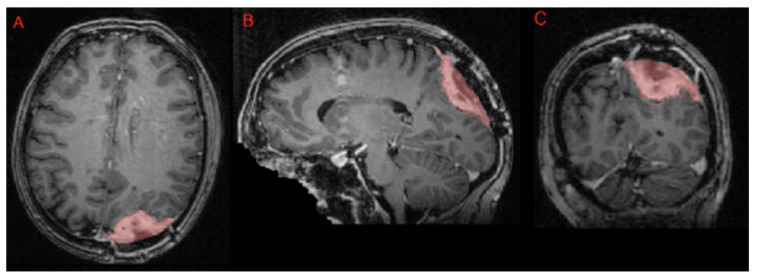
Brain MRI of a patient showing a postoperative left parietal meningioma target volume (red), as delineated by the treating institution, presented in different planes: Axial view in panel (A), sagittal view in panel (B), and coronal view in panel (C). Figure adapted and modified from: [33], licensed under the CC BY 4.0: https://creativecommons.org/licenses/by/4.0/ (accessed 12 October 2024).
